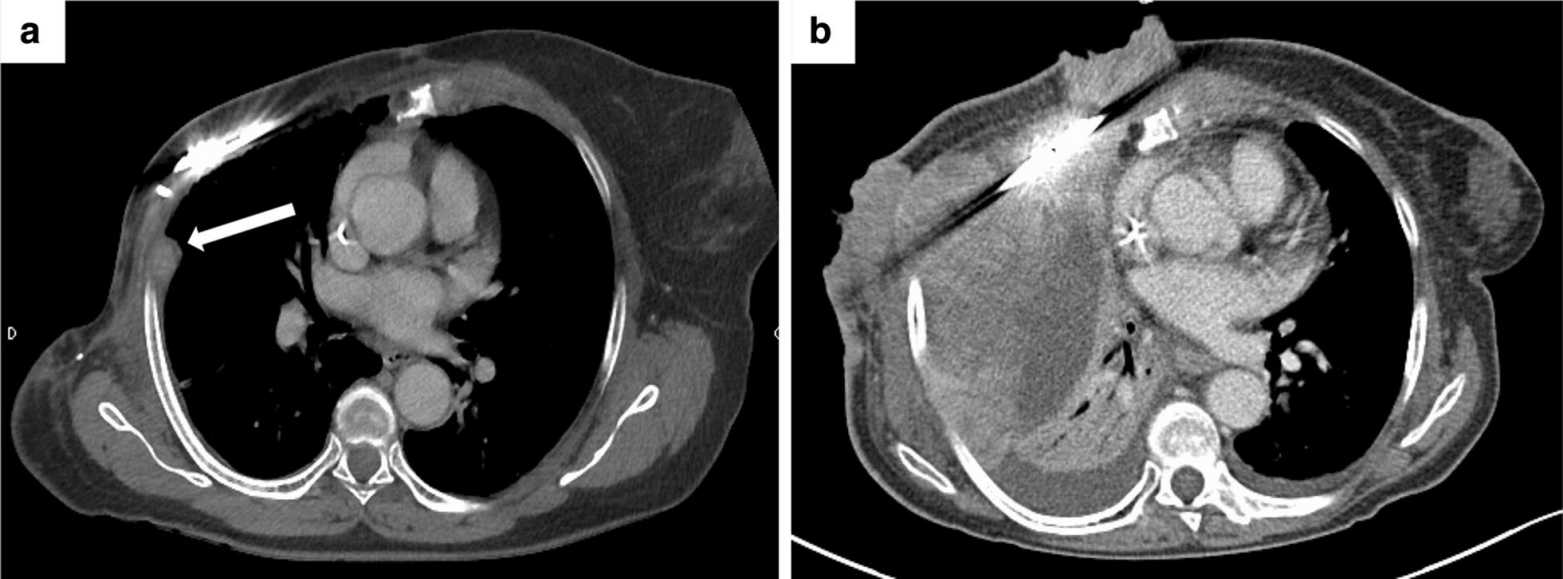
Relapse of the high-grade sarcoma: radiological aspect. a CT-scan in June 2013 showed the appearance of a 2-cm right pleural nodule in the operative field (*white arrow*). The percutaneous biopsy achieved 2 months later will identify high-grade undifferentiated spindle-cell sarcoma. b Major disease progression in September 2014, involving the pleura, the *right* anterior chest wall and the liver
